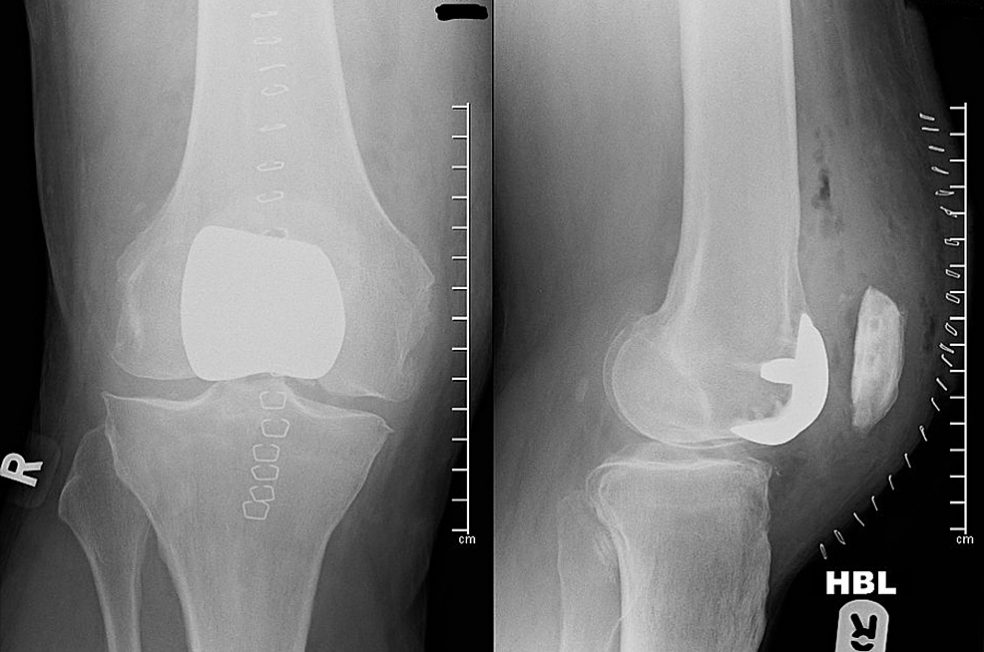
Post-operative radiographs of the right knee following revision of the patella component. The left image is an anteroposterior radiograph of the right knee and the left image a lateral radiograph of the same (non-weight bearing). The patellofemoral joint is congruent following patella revision to an "Onlay Poly" (Microport Orthopaedics) and skin clips are visible over the anterior aspect of the knee.
